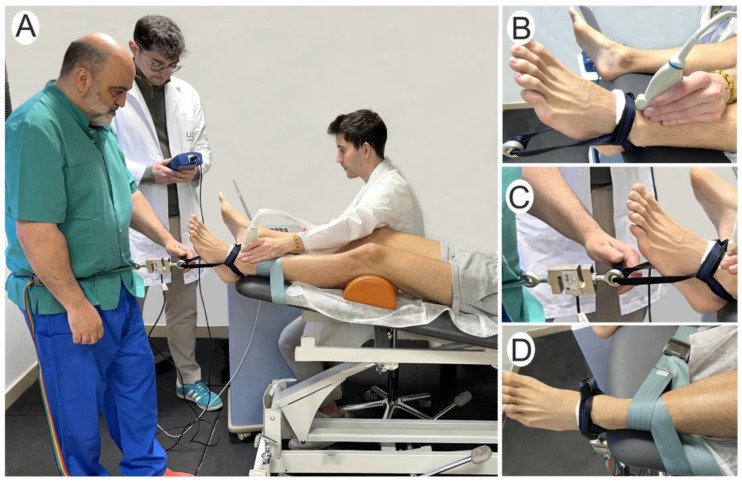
Experimental set-up. (A): Tibiotarsal axial traction technique in fixation condition; (B): ultrasound transducer position; (C): tibiotarsal traction strap and digital force gauge; (D): tibial fixation.
